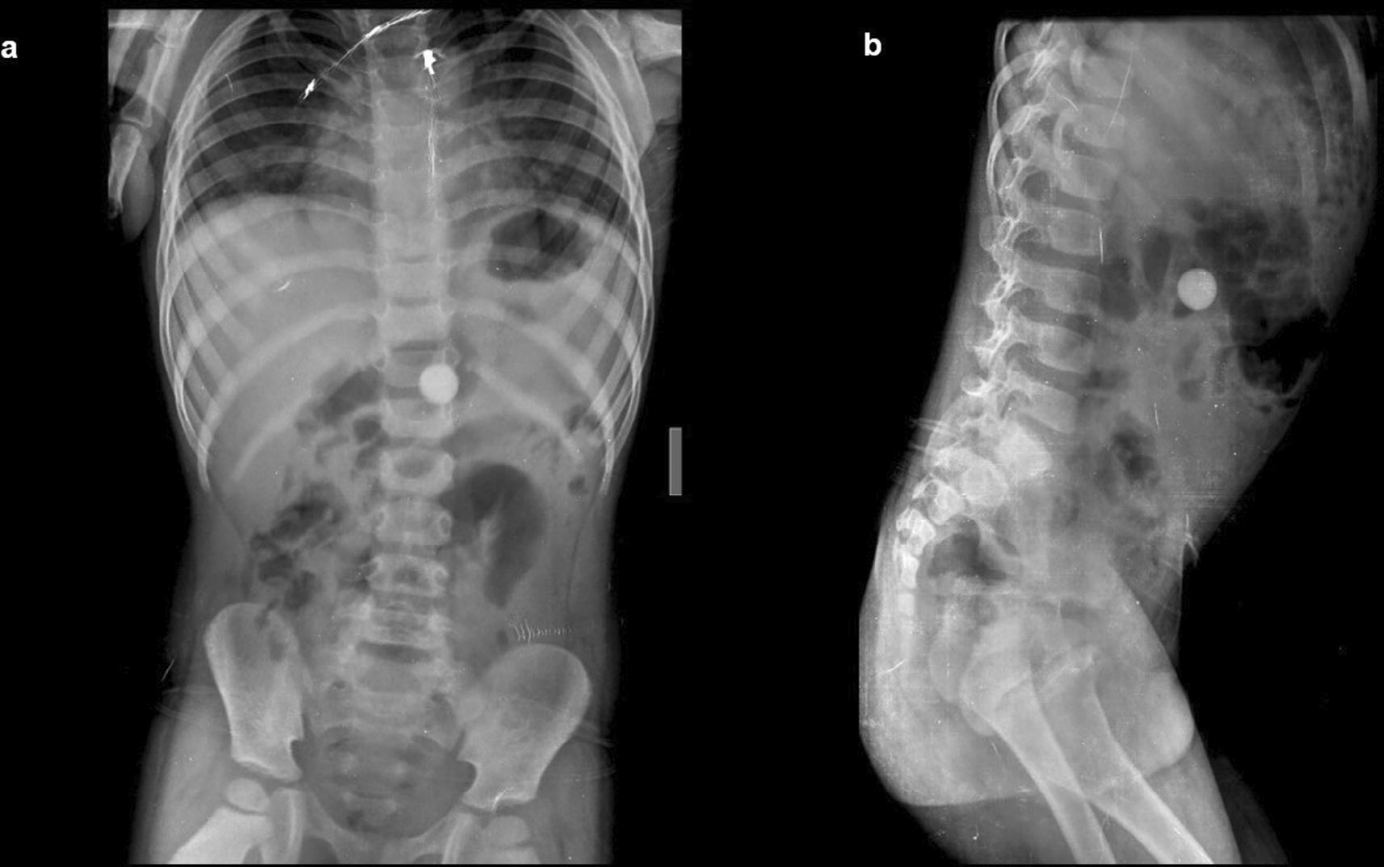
a & b: Radiograph of the abdomen, frontal and lateral projections reveal circular radio opacity on the left side at the level of the L2-L3 disc s/o foreign body likely coin.
